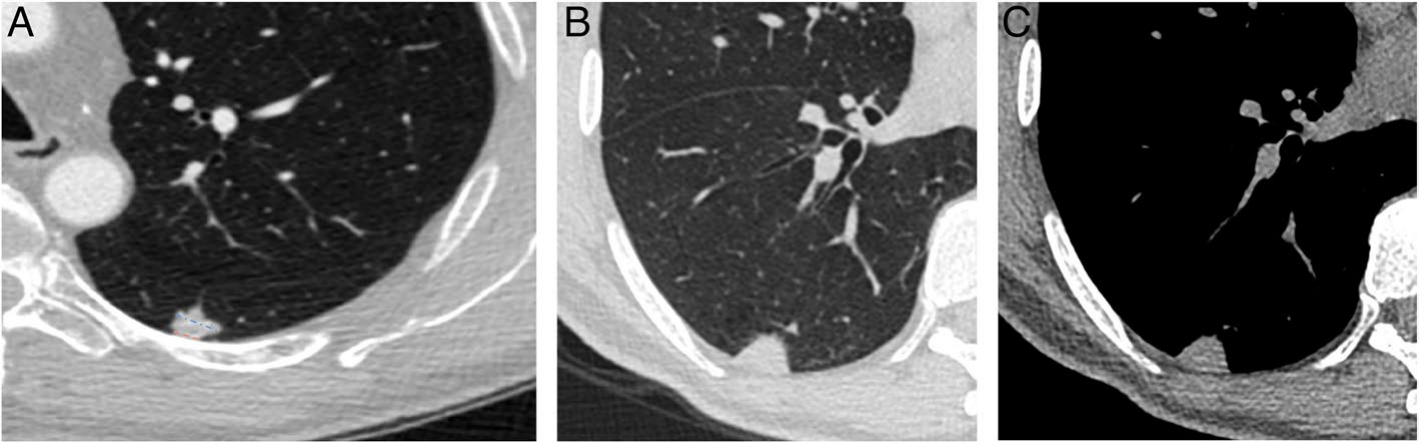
SPAN with narrow or broad basement and type I of nodule-pleura relationship. a A 59-year-old man with left lower lobe lobulated SPAN which is confirmed as invasive adenocarcinoma. On the axial CT image with a maximum section of the nodule, R-length is defined as the ratio of the length of the nodule-pleura interface (red dashed line) to nodule size (blue dashed line). Its R-length is 0.36, which indicates a narrow basement. The nodule-pleura relationship is type I (nodule clinging to the normal pleura without extrapleural fat infiltration). b, c A 49-year-old man with right lower lobe SPAN which is confirmed as a benign lesion during follow-up. On the axial CT image with a maximum section of the nodule, its R-length is 1, which indicates a broad basement, and the nodule-pleura relationship is also type I
